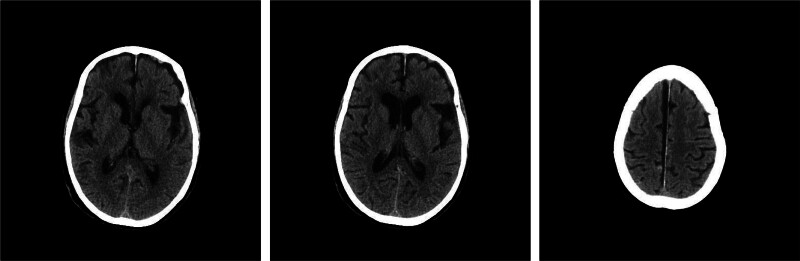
Repeat head CT image after consideration of Guillain–Barré syndrome. CT = computed tomography.
